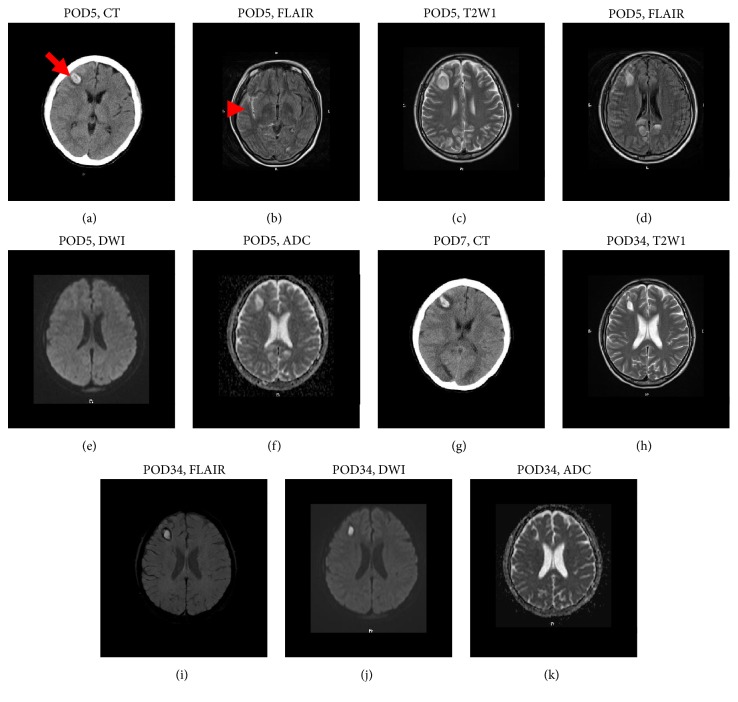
(a) CT image 5 days after delivery, just after the occurrence of tonic-clonic seizure. Arrow indicates intraparenchymal hemorrhage with 2 cm diameter in the right frontal lobe. (b) FLAIR image on MRI 5 days after delivery, just after the occurrence of tonic-clonic seizure. Arrowhead indicates subarachnoid hemorrhage at the right Sylvian fissure. (c) T2-weight image on MRI 5 days after delivery, just after the first seizure. (d) FLAIR image on MRI 5 days after delivery. (e) DWI on MRI 5 days after delivery. (f) ADC mapping on MRI 5 days after delivery. (g) CT image 7 days after delivery, just after the second tonic-clonic seizure. (h) T2-weighted image on MRI 34 days after delivery. (i) FLAIR image on MRI 34 days after delivery. (j) DWI on MRI 34 days after delivery. (k) ADC mapping on MRI 34 days after delivery. CT, computed tomography; MRI, magnetic resonance imaging; POD, postoperative days; T2W1, T2-weighted; FLAIR, fluid-attenuated inversion recovery; DWI, diffusion-weighted image; ADC, apparent diffusion coefficient.
